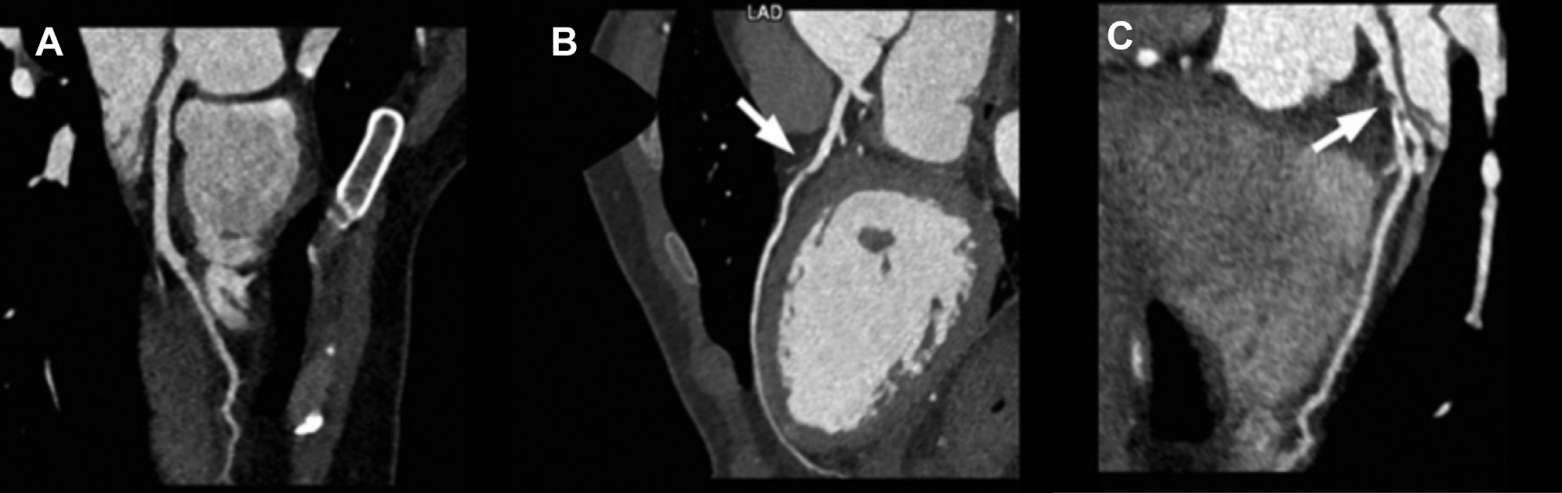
Representative cardiac computed tomography angiography images of the coronary arteries. (A) Curved planar reformation (CPR) demonstrating no evidence of coronary artery disease in the left anterior descending artery (LAD). (B) CPR demonstrating 50% stenosis of the LAD due to noncalcified plaque. (C) Multiplanar reformation demonstrating > 70% stenosis of the LAD due to noncalcified plaque.
